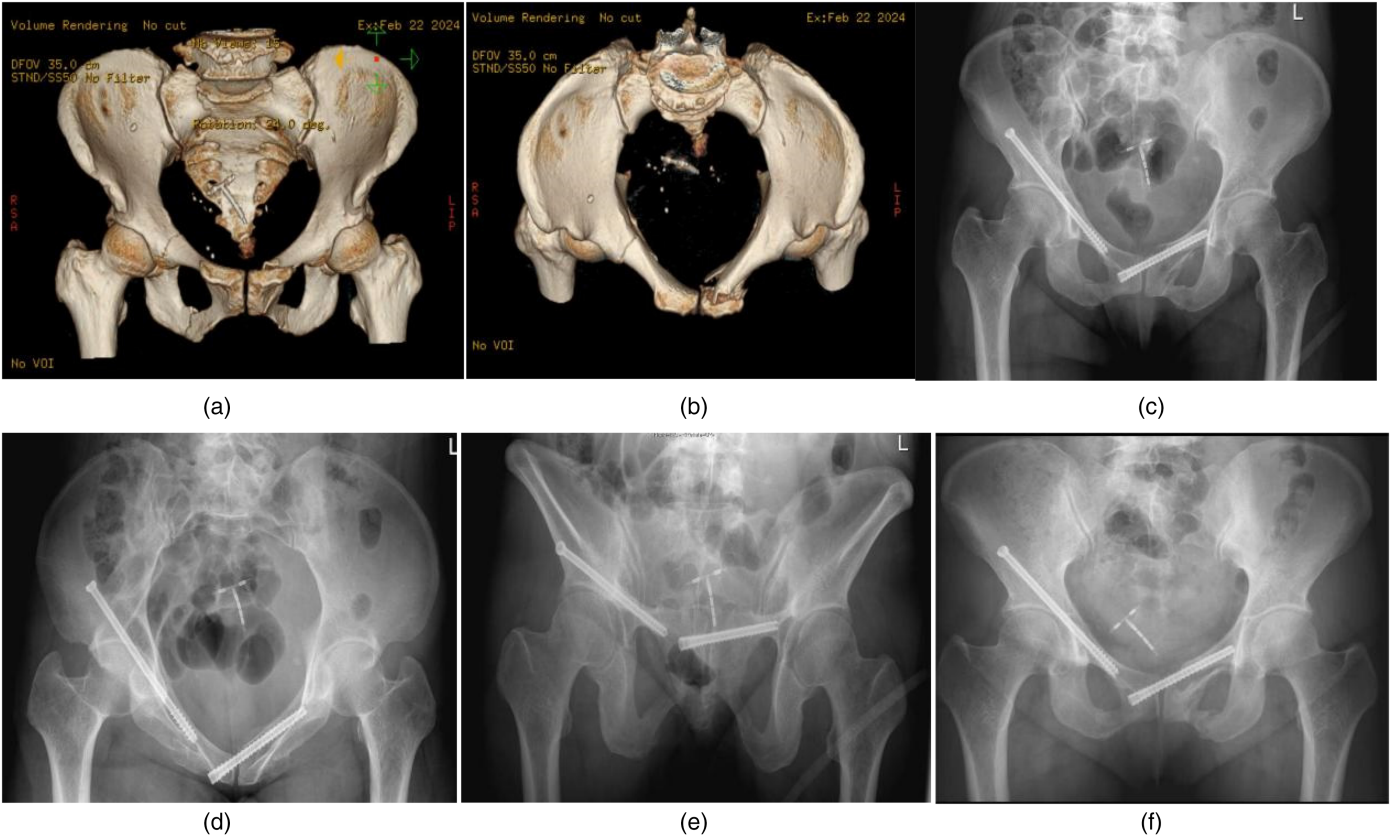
(a) Preoperative 3D CT anterior view: Bilateral anterior pelvic ring fractures including left symphysis pubis fracture. (b) Preoperative 3D CT entrance view: The integrity of the pubic symphysis was destroyed. (c) Screw position as shown in postoperative pelvic anteroposterior view. (d) Screw position as shown in the postoperative pelvic entrance view. (e) The screw position as shown in the postoperative pelvic outlet view. (f) Anteroposterior pelvic images at 3 months postoperatively showed callus formation and no screw loosening.
